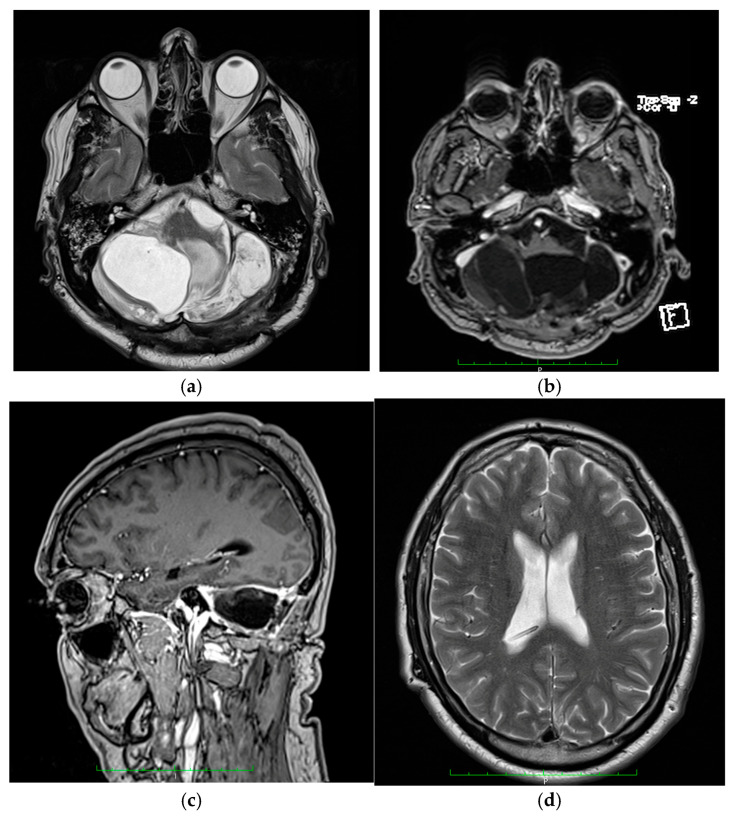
MRI results of patient one after surgery. (a) Axial section showing post-resection cystic cavity in the left cerebellar hemisphere. (b) Axial section, postcontrast T1 sequence, highlighting complete tumor resection. (c) Sagittal section, postcontrast T1 sequence. (d) Axial section, T2 sequence, which reveals a normal Taline ventricle system with a ventricular catheter placed at the level of the right ventricular atrium; the patient developed hydrocephalus therefore he was operated on and had a ventriculo-peritoneal drainage performed. All four frames were taken during the same MRI exam.
